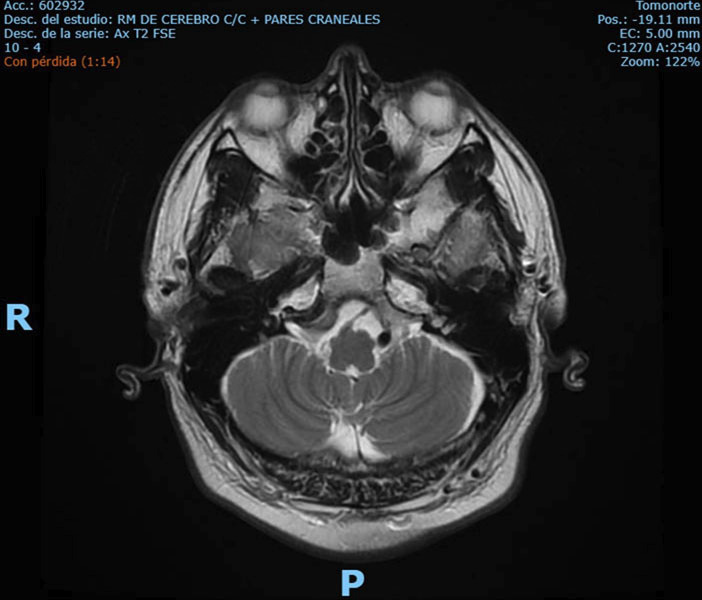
Brain MRI in axial T2 sequence showing skull base and cerebellopontine angle structures without evident lesions.
